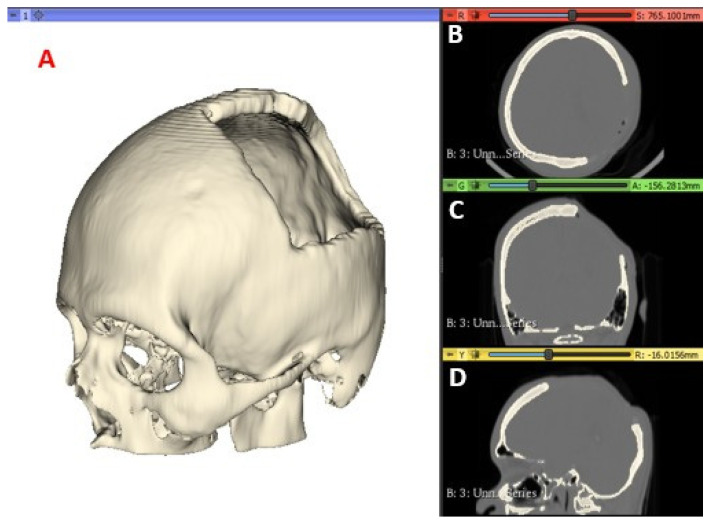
CT Scan Images: segmented model (A); axial view (B); coronal view (C); and sagittal view (D).
